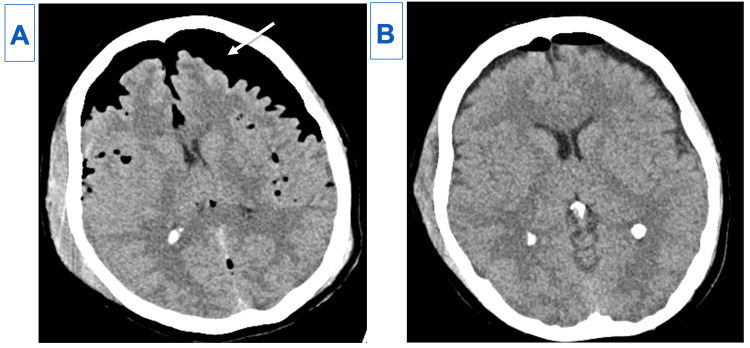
(A) Follow-up computed tomography (CT) the next AM showing tension pneumocephalus with the classic Mount Fuji sign (arrow). (B) 72 hours post-treatment CT showing near resolution of tension pneumocephalus.
